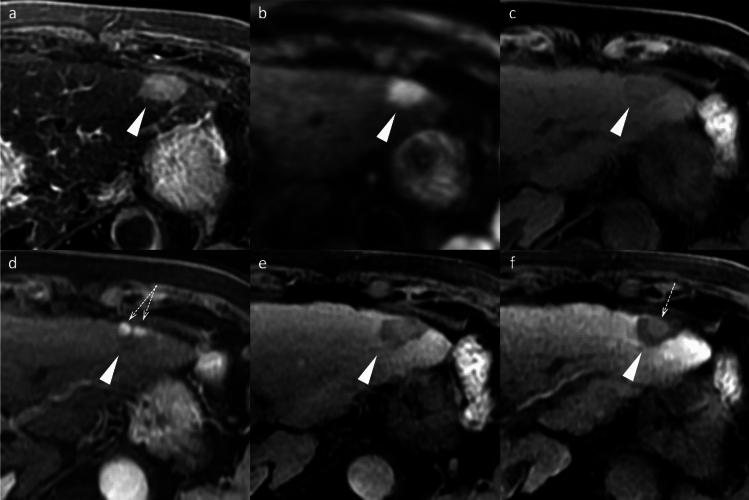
Hepatocellular carcinoma with a nodule-in-nodule appearance in an 82-year-old woman. A nodule (arrowheads) in segment 3 displays homogeneous hyperintensity on fat-suppressed T2-weighted imaging (a) and homogeneous diffusion restriction on diffusion-weighted imaging (b). The nodule appears hypointense relative to the background liver on the pre-contrast image (c). In the arterial phase (d), a portion of the nodule exhibits hyperenhancement, consistent with a nodule-in-nodule appearance (arrows). The nodule appears hypoenhancing relative to the background liver on the transitional phase image (e), and the nodule-in-nodule appearance is again evident on the hepatobiliary phase image (arrow) (f)
